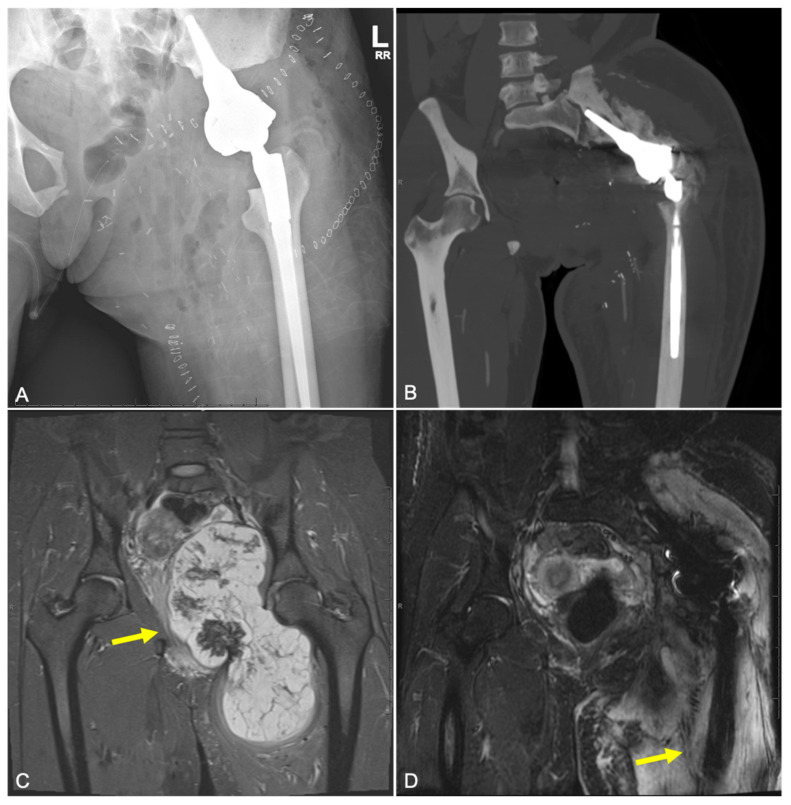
(A) Radiograph and (B) CT image of 47-year-old female patient with history of pelvic chondrosarcoma treated with internal hemipelvectomy and endoprosthetic reconstruction, now experiencing infection 2 months post-operatively (type 4A failure), requiring debridement of left hip wound. Patient previously experienced a type 1B failure, aseptic wound dehiscence, before deep infection occurred. (C) MR image of the original tumor (yellow arrow), measuring 20 cm in longest axis. (D) MR image demonstrating exposed, sclerotic left iliac bone (yellow arrow) consistent with chronic osteomyelitis as well as possible hardware loosening with fluid surrounding prosthesis stem.
